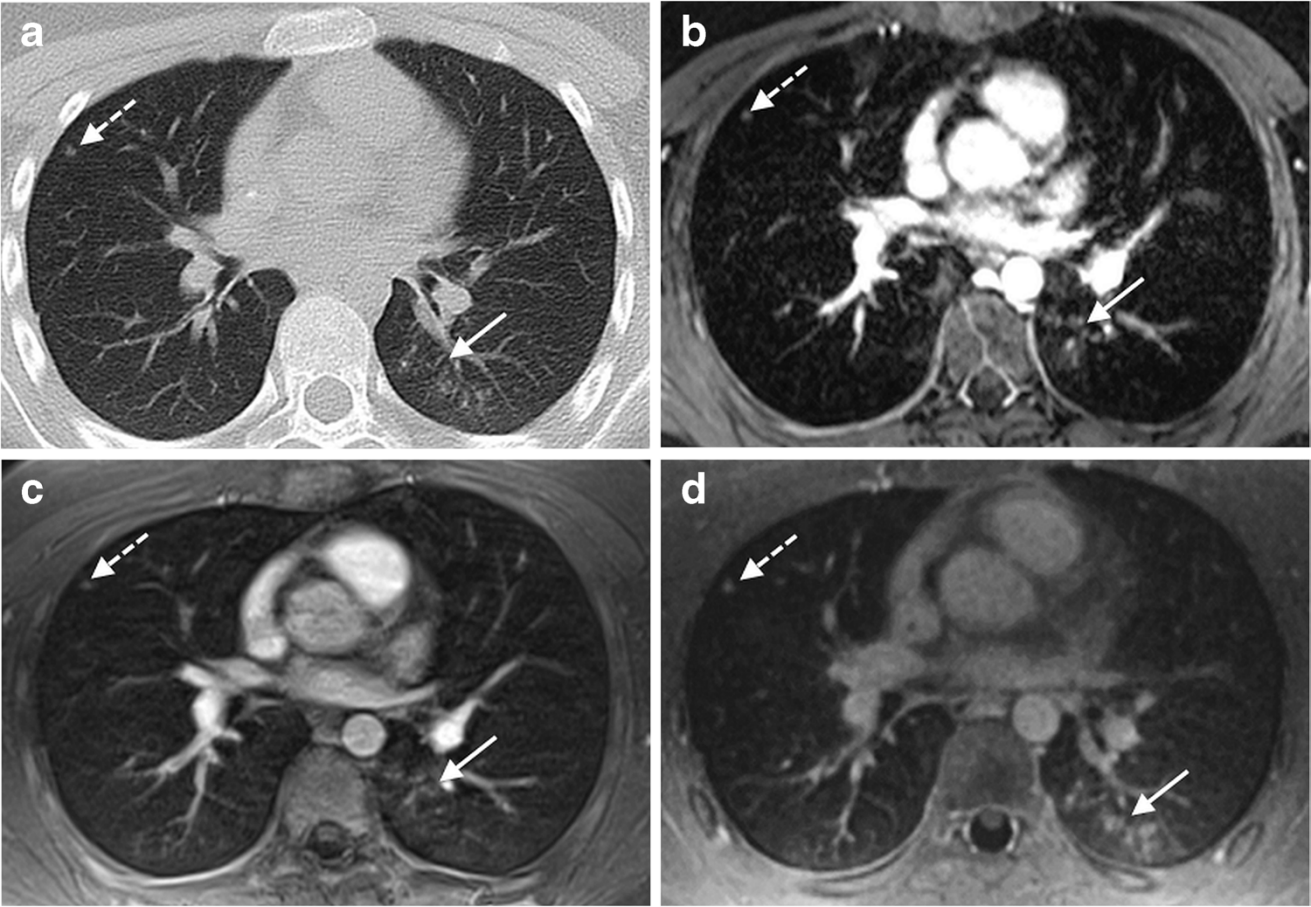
A 14-year-old male patient with Ewing’s sarcoma and aspergillosis. Pulmonary nodule (axial average diameter of 4 mm) in the right middle lobe was detected on CT and on all T1-weighted MR sequences (discontinuous arrows). The aspergillosis infection presented as ground-glass opacity with some nodular pattern in the left lower lobe (continuous arrow). The pathology was diagnosed on chest CT (a). With the incoherent GRE (b) and the volume interpolated GRE sequence (c), the infection was not detected during the initial analysis as it was misinterpreted as hypostasis and artefacts (continuous arrows). However, the ground-glass opacity was observed in the second-look sessions of both sequences in comparison with CT. In the UTE image (d), the pathology was correctly diagnosed during the initial evaluation session (continuous arrow)
